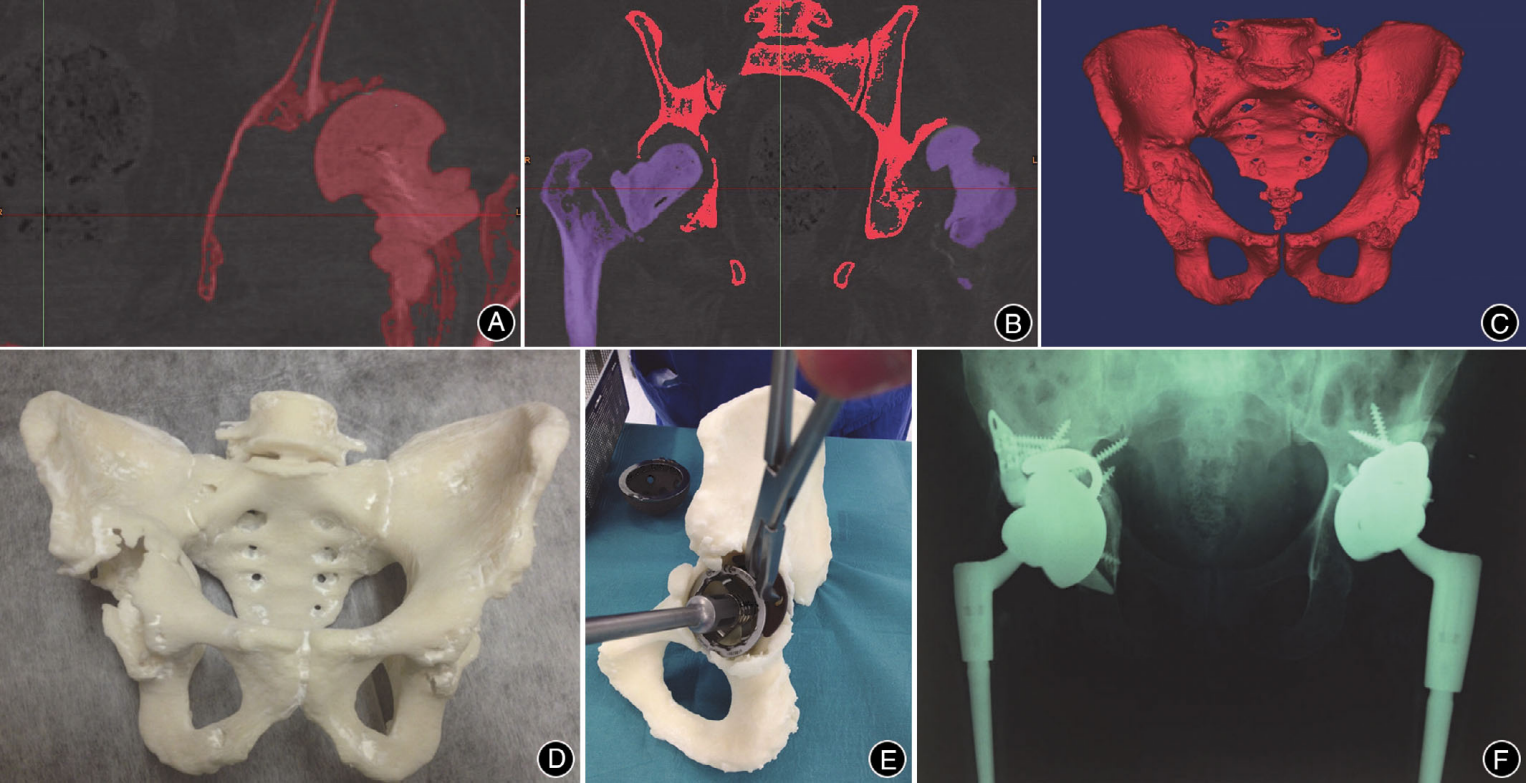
A, Image thresholding was performed by using software, which allowed for bone to be differentiated from surrounding soft tissue based on bone and soft tissue densities on the CT scan; B, Using the region growing process, both femurs were digitally segmented from their corresponding pelvis. The red pelvis will be retained, while the purple femurs will be removed; C, Once both femurs were erased, the 3D isolated image of pelvis (namely, the anatomy of interest) was created; D, The final life‐size 3D‐printed pelvis model, providing the surgeon with visual and tactile appreciation of the defects; E, Acetabular cup, augment, and buttress sizes, as well as cage dimensions were selected and trialed in preoperative surgical stimulation using a 3D‐printed pelvis; F, Postoperative anteroposterior pelvic plain film radiographs showed satisfactory revision total hip arthroplasty *in situ*
